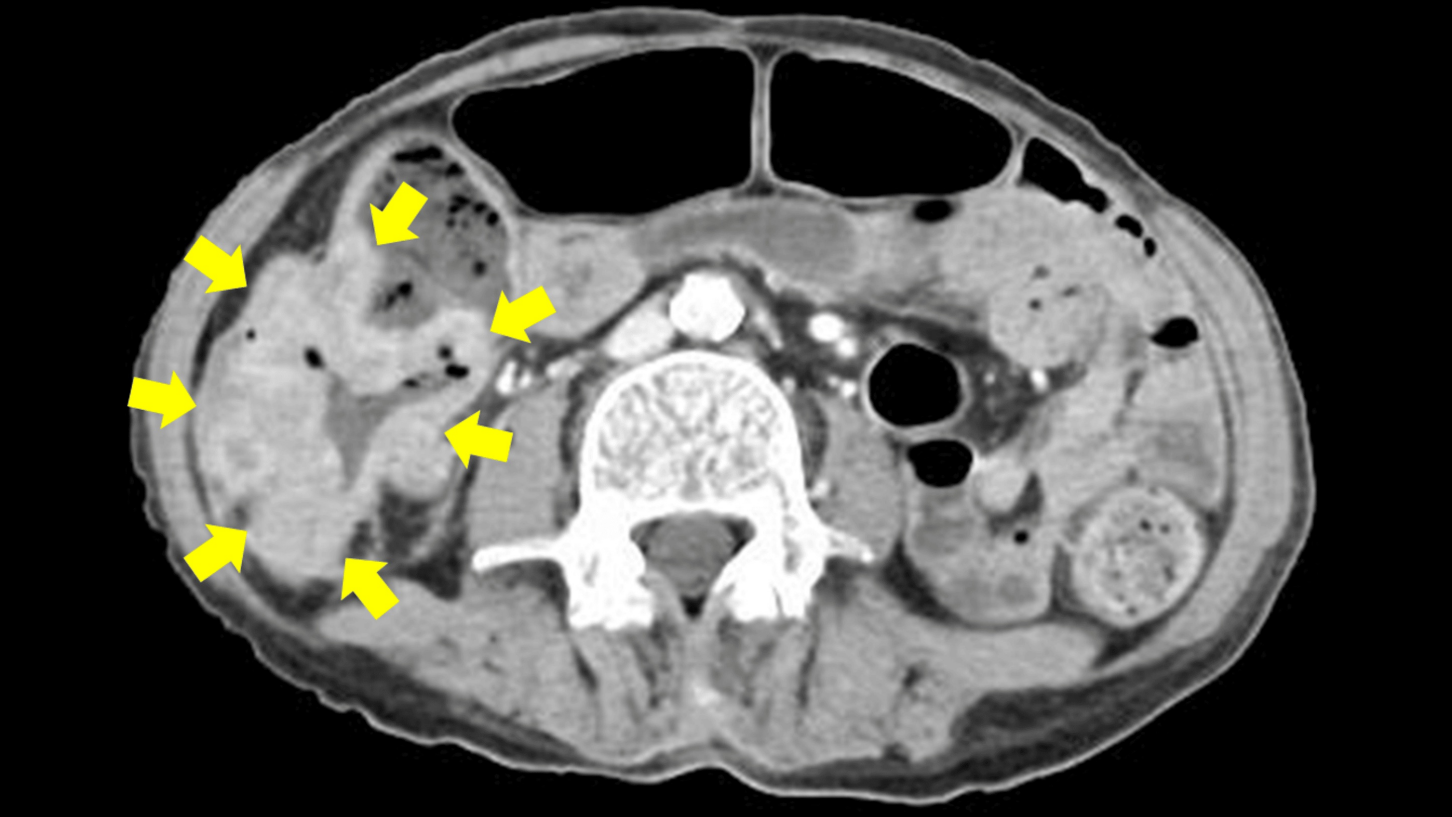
Contrast-enhanced abdominal CT demonstrating ascending colon tumor. The axial CT image shows circumferential wall thickening with irregular enhancement in a portion of the ascending colon (arrows).
